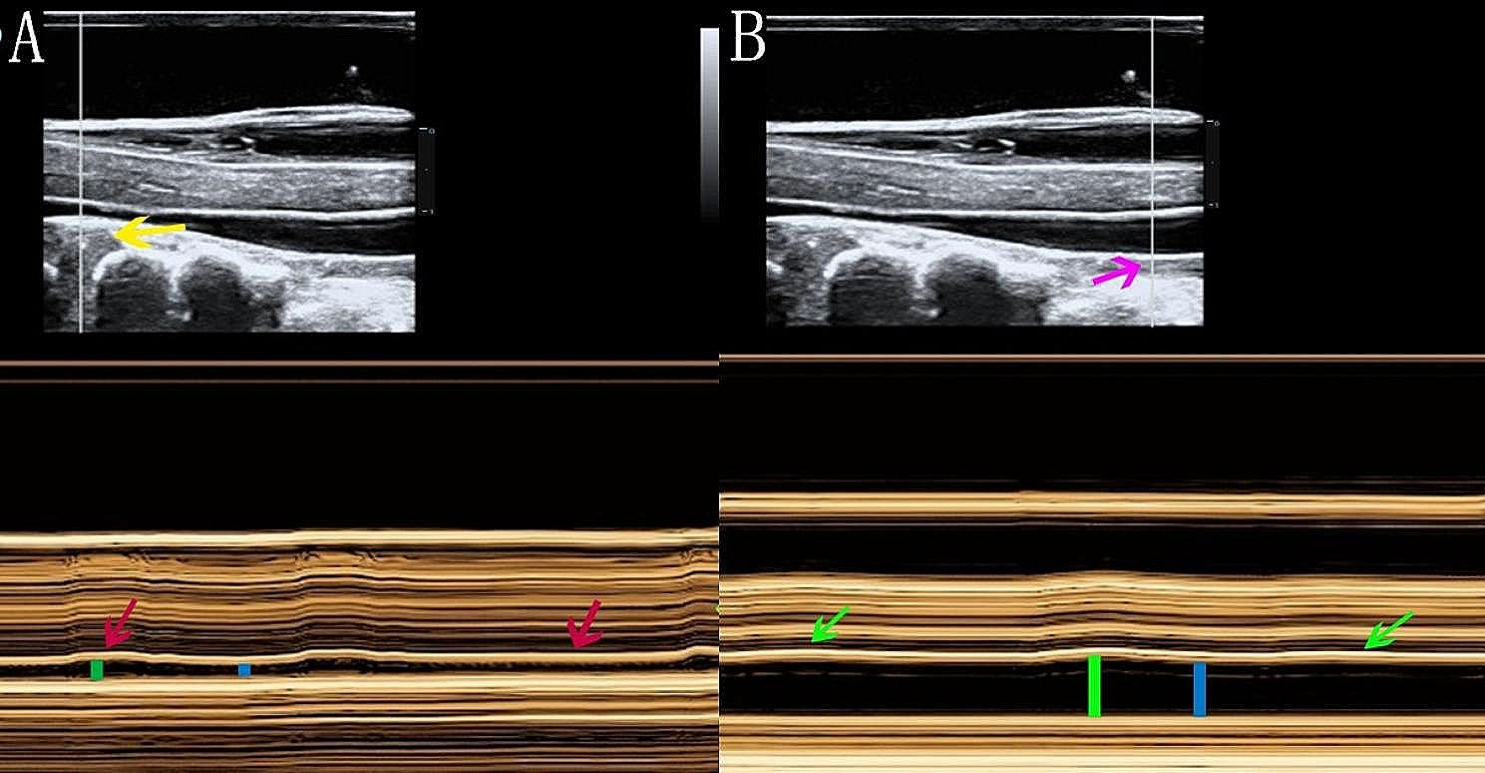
SCPA in the CA (A) and NCA (B) visualized via M-mode ultrasound. (A) Sample line passing through the CA (yellow arrow). (B) Sample line passing through the NCA (pink arrow). The wavy lines graphically depict the SCPA in the CA (red arrow) and NCA (green arrow). SCPA = length of the green line minus the length of the blue line. The distance from 0 to 1 on the scale represents 1 centimeter
